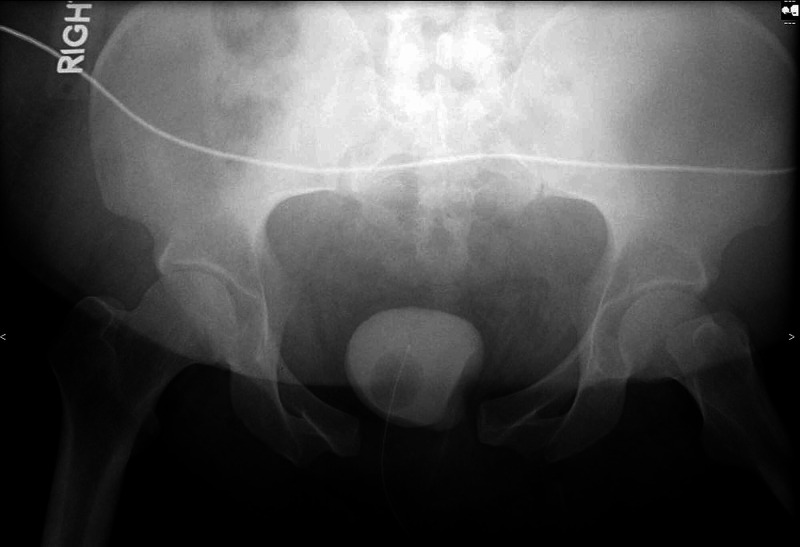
AP pelvis injury radiograph demonstrating APC type II pelvic ring injury. AP: anteroposterior; APC: anteroposterior compression
